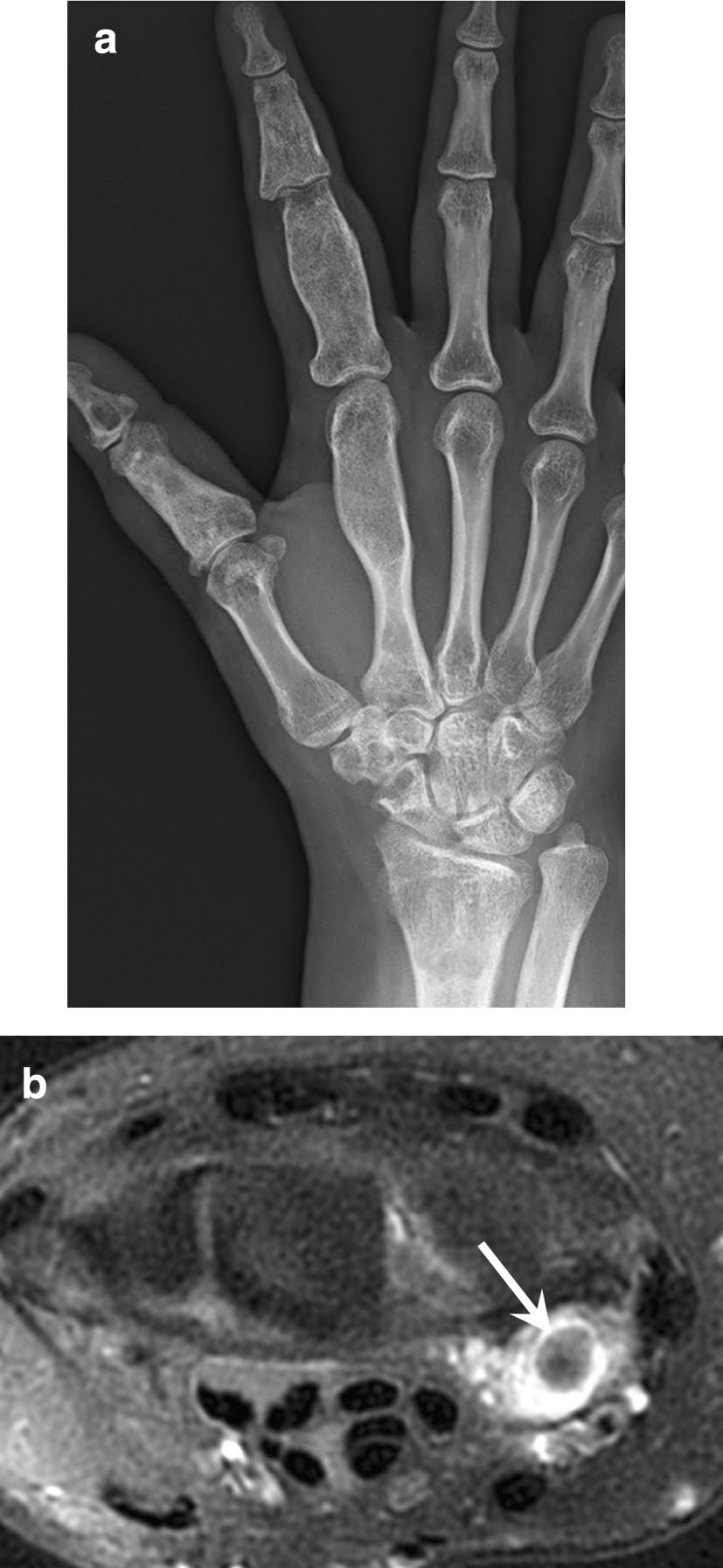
Maffucci syndrome in a 36-year-old woman. (a) Standard radiography showing the typical multiple enchondroma of the bones of the hand. (b) Axial T2-weighted MR image showing a haemangioma characterised by cavernous vascular spaces containing phleboliths (arrow)
